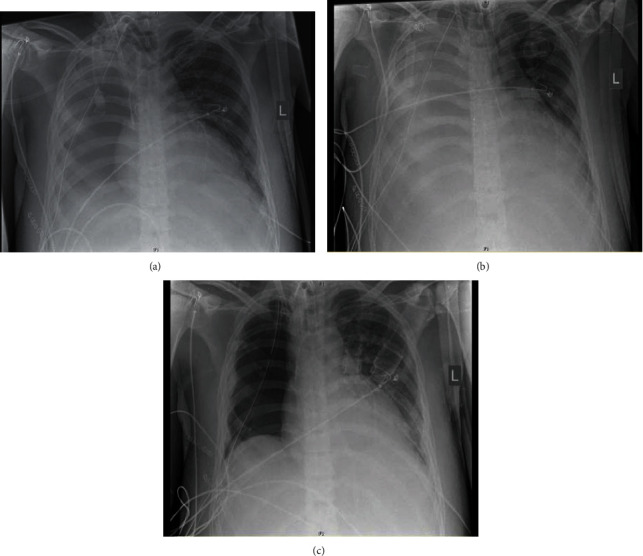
Series of chest X-rays during the time of the VATS operations.
